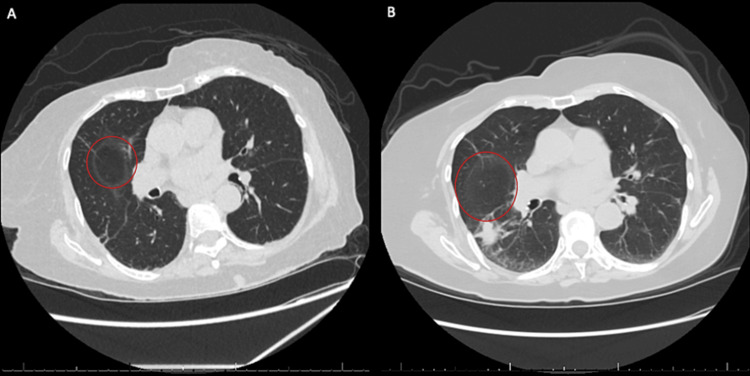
CT chest without contrast demonstrating pulmonary findings of the right lower lobe Cavitary lesion denoted with red circles. (A) CT from current admission showing interval development of cavitary lesion. (B) CT chest from previous admission one week prior with right lower lobe consolidation.
